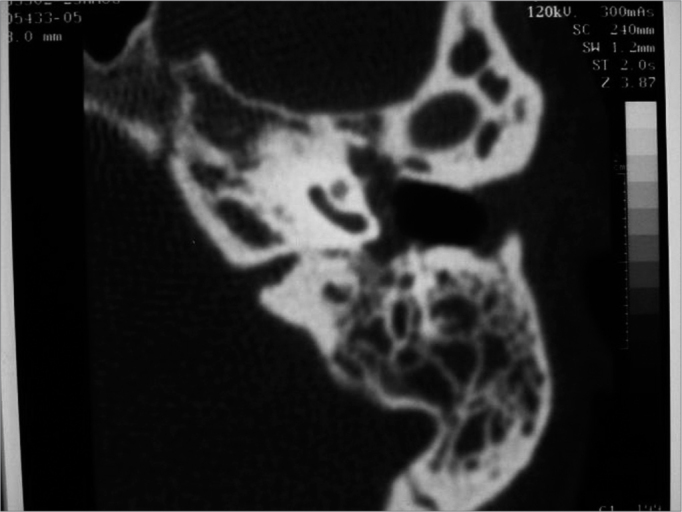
Case 2. Computed tomography showing treatment-resistant otomastoidits. Plain mastoidectomy was recommended.
